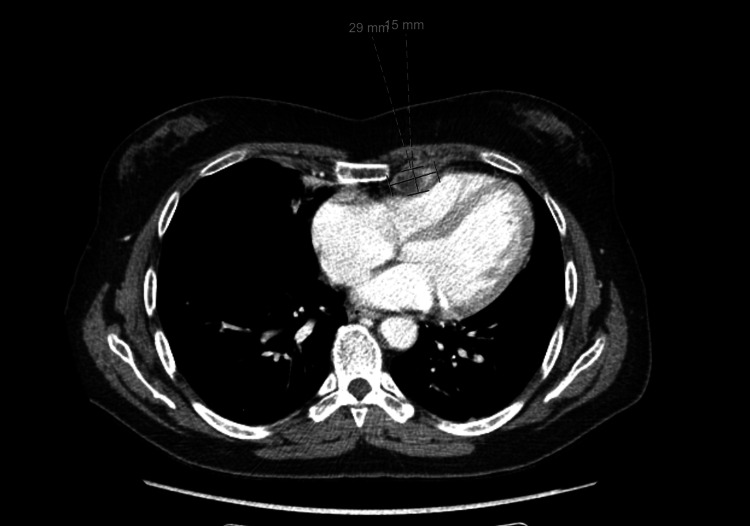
Axial CT showing necrotic-appearing lymph nodes in the left internal mammary chain
